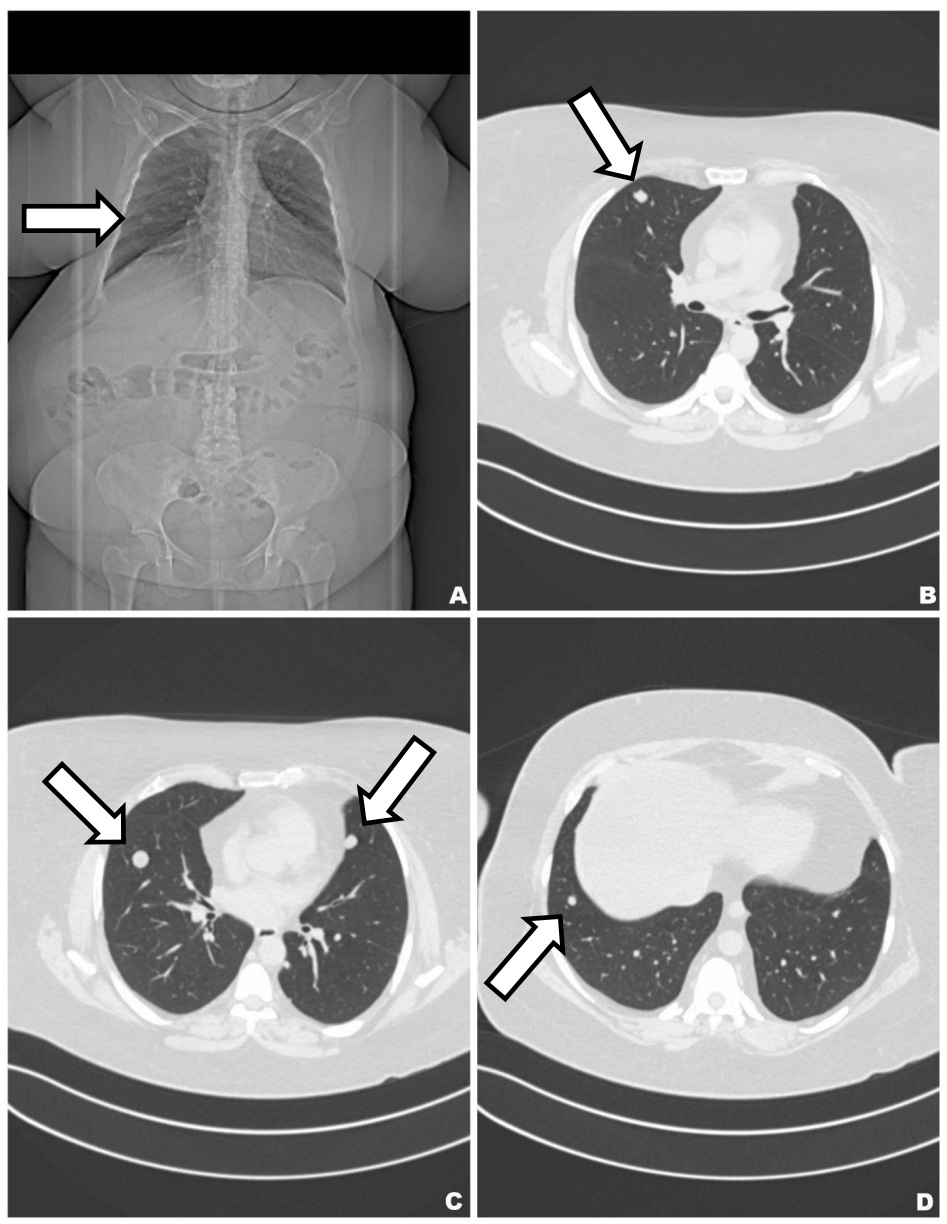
CT of the lung. Multiple cystic nodules are present in both lungs (arrows) (A–D).
